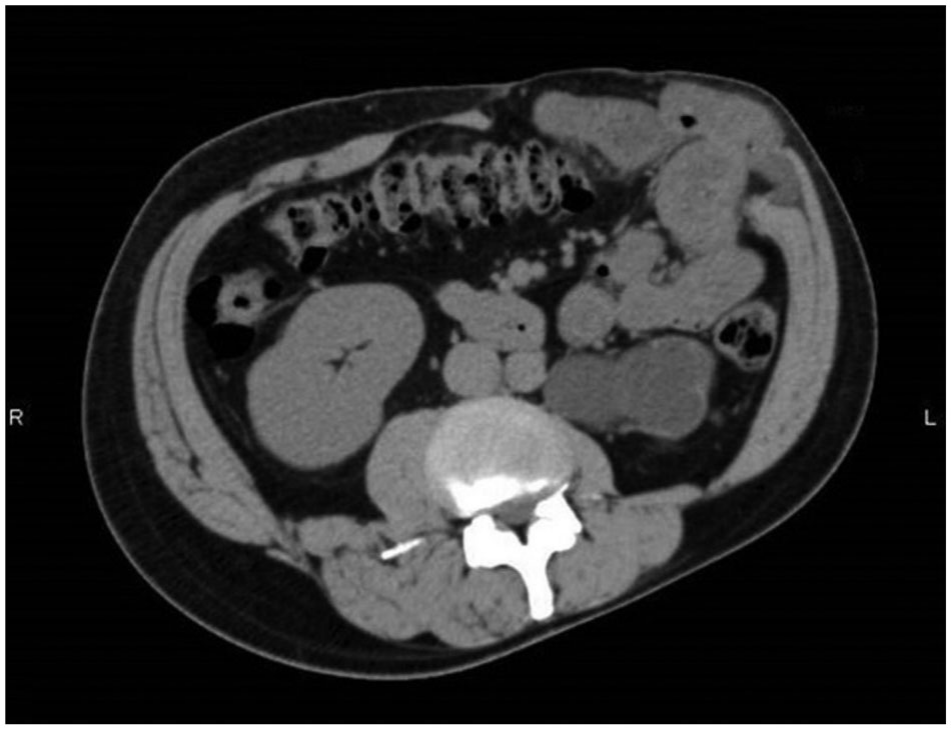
Axial CT image of the abdomen. It shows abdominal wall defect and the herniated small bowel and colon.
